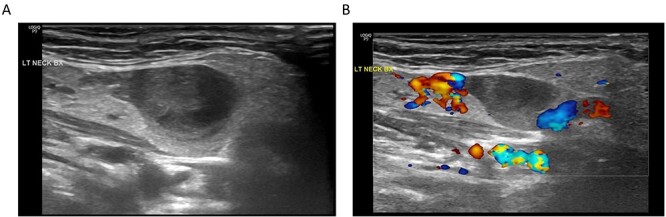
(A) Ultrasonography of the submandibular neck lesion; (B) ultrasonography of the lesion with color Doppler; the apparent lack of flow within the venous aneurysm is likely related to the imaging technique and relatively slow flow within the aneurysm compared to the adjacent external jugular vein.
